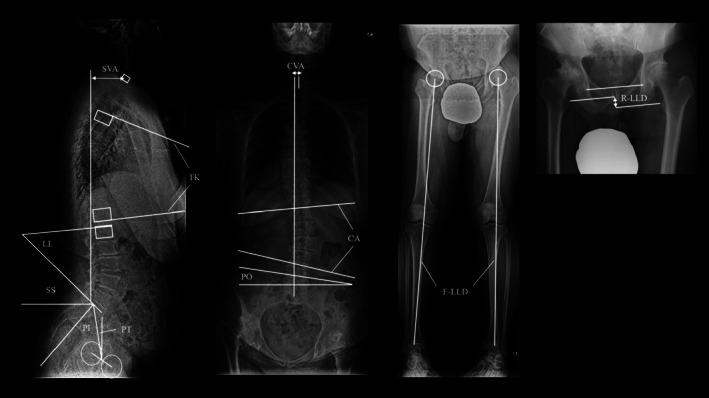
Radiographic parameters. CVA, coronal vertical axis; F‐LLD, functional leg length discrepancy; LL, lumbar lordosis; PI, pelvic incidence; PO, pelvic obliquity; PT, pelvic tilt; R‐LLD, radiographic leg length discrepancy; SS, sacral slope; SVA, sagittal vertical axis; TK, thoracic kyphosis.
